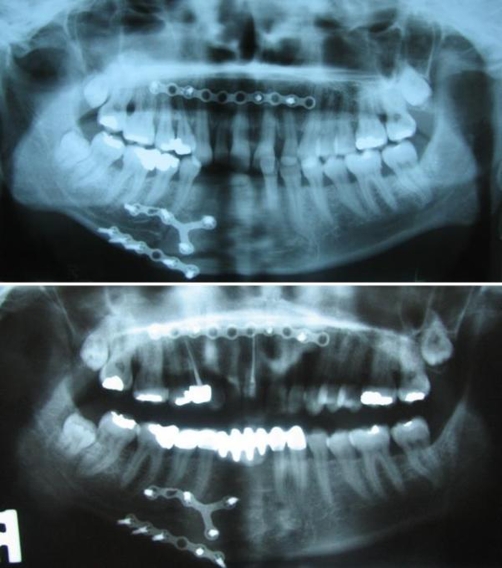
The radiographic examination indicates the superior position of the maxillary anterior segment to the occlusal plane.
